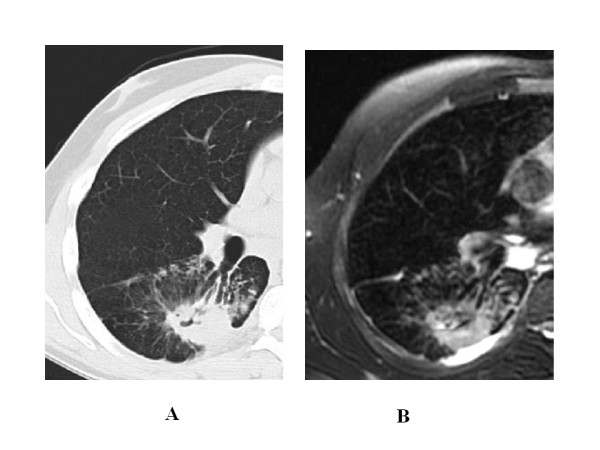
61 year-old woman with pulmonary tuberculosis. A) HRCT shows cavited infiltrate. B) Unenhanced MRI identifies the same features as well as HRCT and identifies subtle, free pleural effusion, and a highly hyper-intense mediastinal lymph node.
